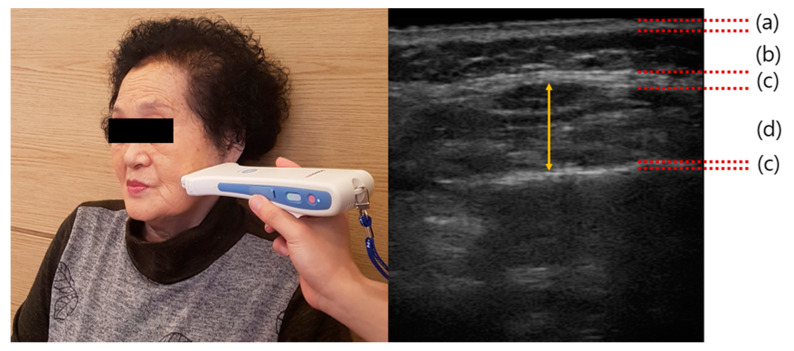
Measurement of masseter muscle thickness. Left panel, image recorded during the actual measurement of masseter muscle thickness and right panel, ultrasound image showing the anatomical structure of the masseter muscle. (a) Skin layer; (b) Fat layer; (c) Masseteric fascia; and (d) Masseter muscle.
